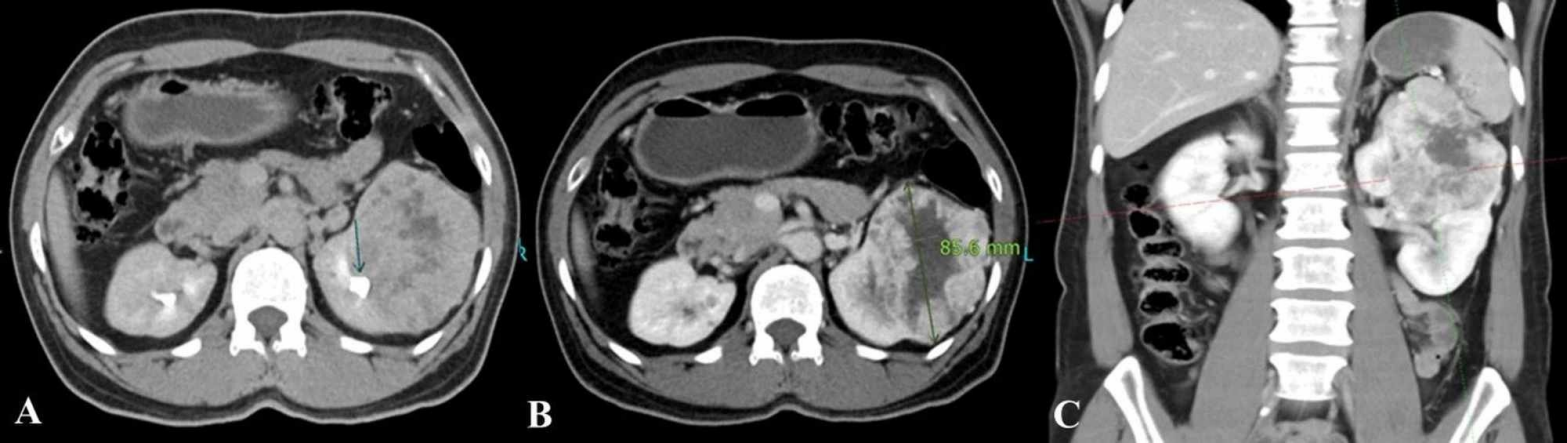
High complexity lesion (score 11x) according to the RENAL nephrometry score A) Excretory phase of the triphasic CT scan showing a left renal lesion abutting the renal collecting system. B) Venous phase axial CT showing the same lesion diameter of 8.5 cm. C) Venous phase coronal reformatted image shows <50 % of the lesion being exophytic and crossing the axial midline. RENAL: radius, exophytic/endophytic properties, nearness of tumor to the collecting system or sinus in millimeters, anterior/posterior, location relative to polar lines
